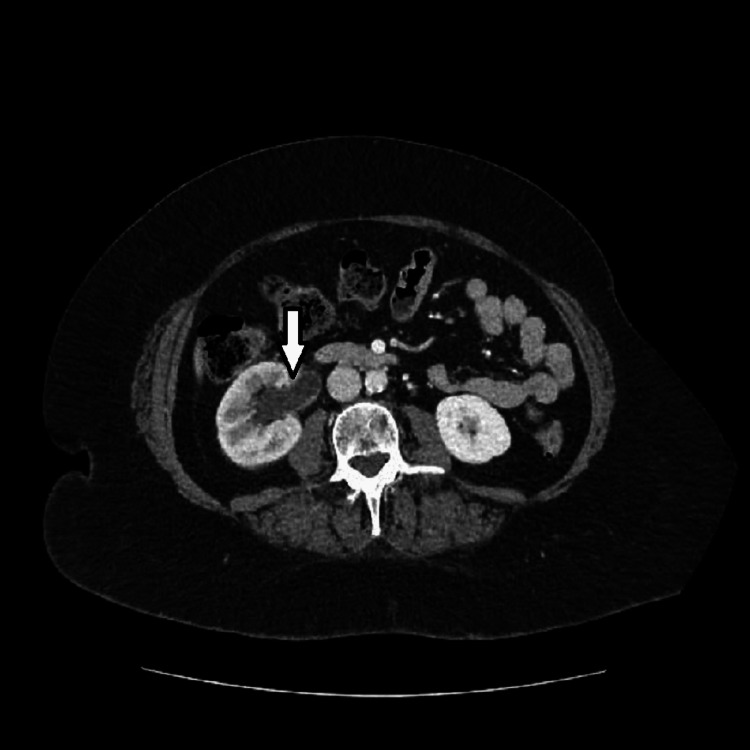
CT scan showing right-sided hydronephrosis
